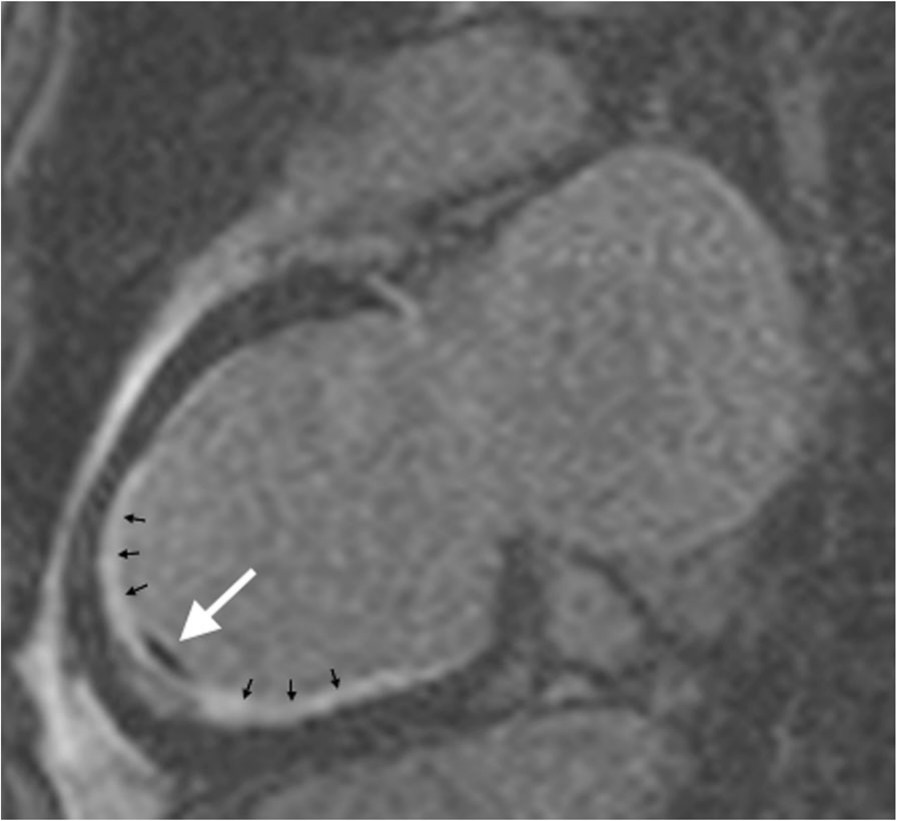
Cardiac magnetic resonance imaging (1.5 Tesla, 2 chamber view, late gadolinium enhancement, inversion recovery, inversion time [TI]: 190 ms). Cardiac magnetic resonance imaging showing extended semicircumferential subendocardial late enhancement indicating endomyocardial fibrosis (small black arrows) and an apical thrombus (long white arrow)
